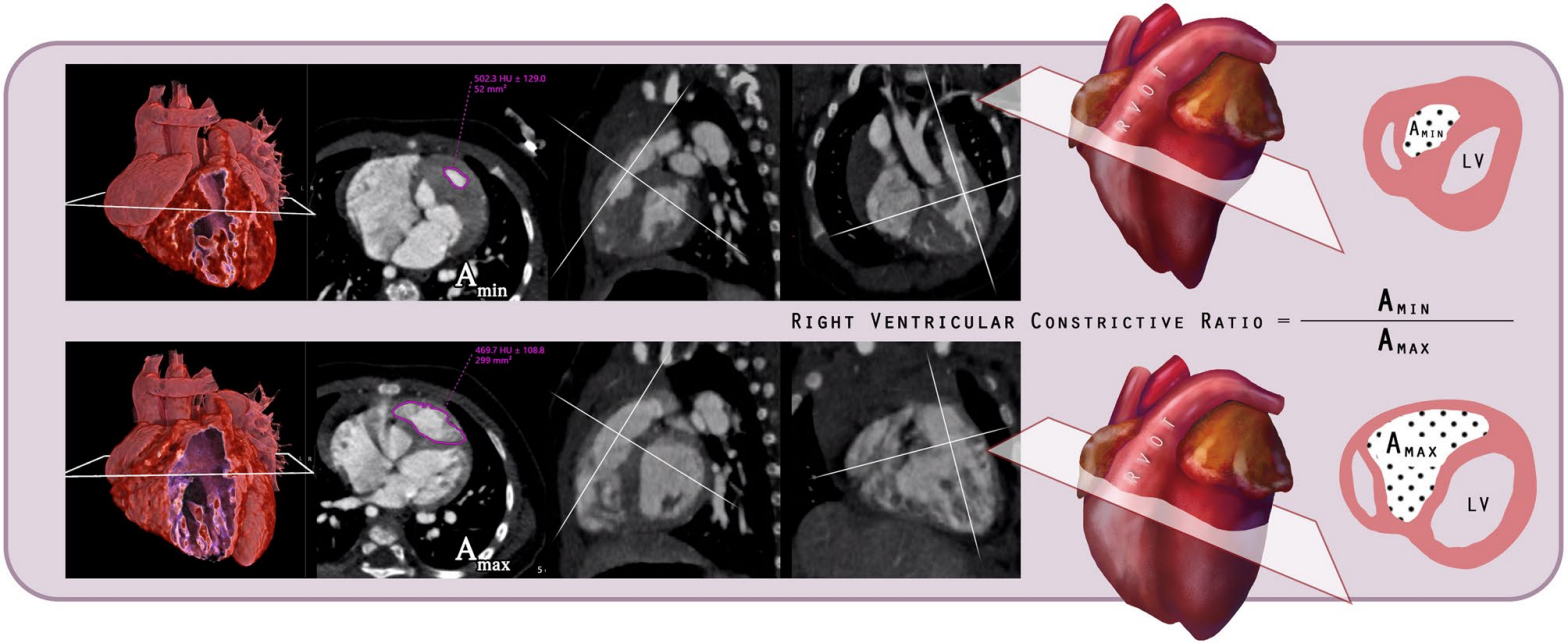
The patient was diagnosed with simple VSD and DCRV via CT. Surgeons performed a patch repair for VSD and resection of the hypertrophied muscular bundle. Three-dimensional myocardial model images of his heart during the end-systole (upper row) and end-diastole (lower row) are shown. The reformatted CT images from left to right are oblique axial, oblique sagittal, and oblique coronal sections in both upper and lower rows. Measured luminal areas A_min_ and A_max_ are outlined (in pink) on the cross-sectional images paralleled to the apparent muscular ridge. Right ventricular constrictive ratio (A_min_/A_max_) for this patient was 17.4%. [*CT *computed tomography; *DCRV* double-chambered right ventricle; *VSD *ventricular septal defect].
